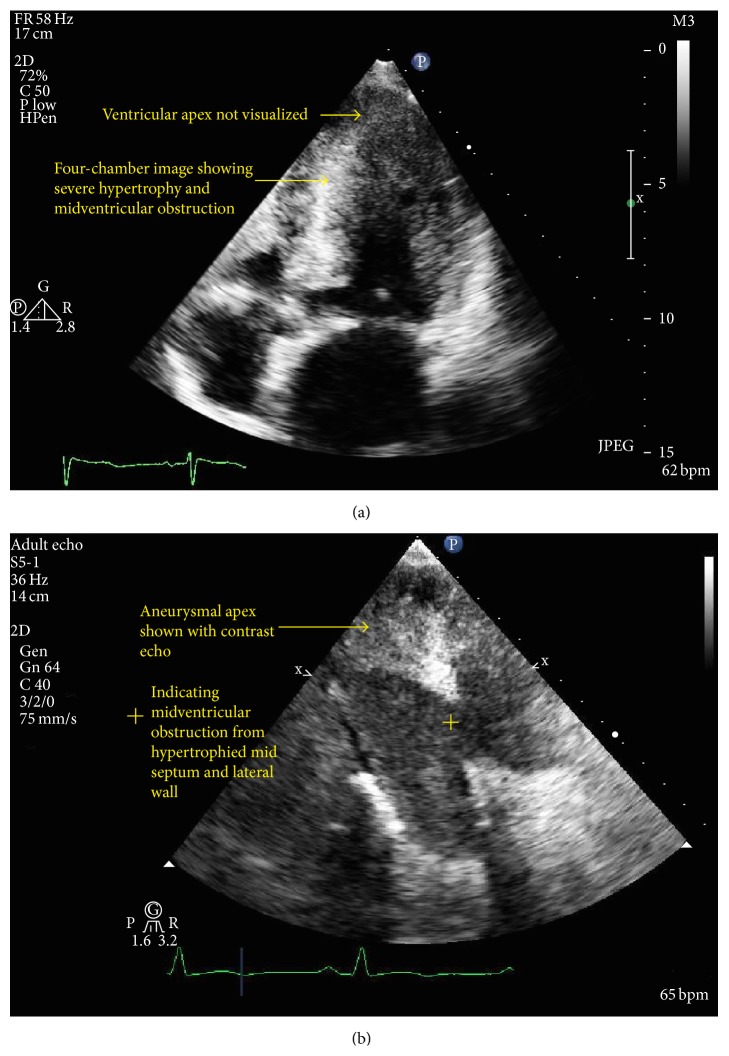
(a) Noncontrast transthoracic echocardiographic 4-chamber image demonstrating left ventricular hypertrophy and inability to visualize apical pouch. (b) Contrast transthoracic echocardiographic image demonstrating midventricular narrowing with aneurysmal apex.
